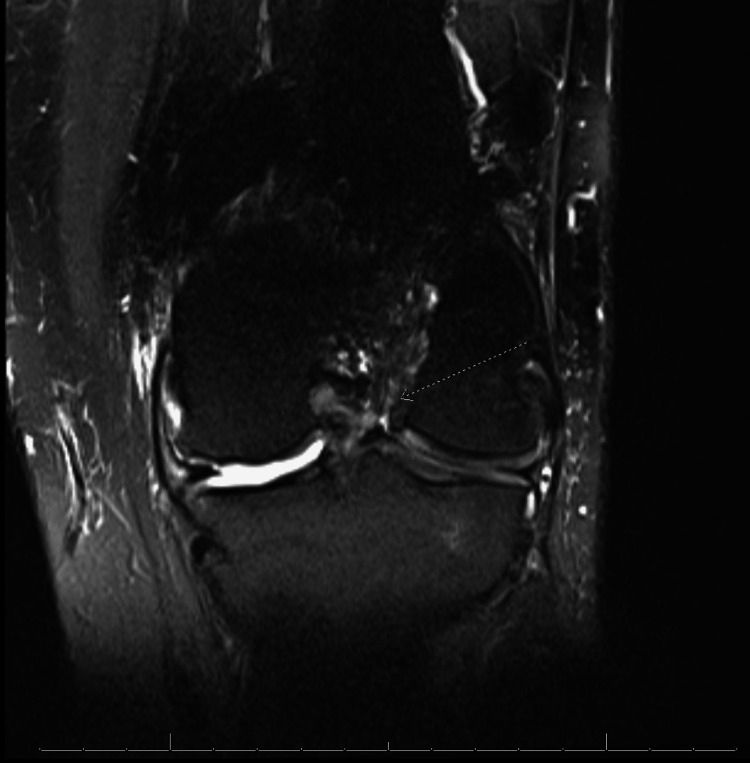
MRI of left knee, anteroposterior view showing previous ACL graft with signal changes concerning for a graft tear (arrow). ACL: anterior cruciate ligament
